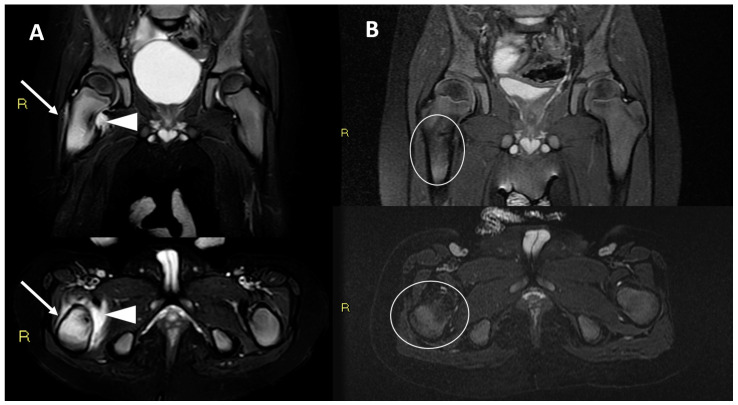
T2w fat saturated MRI images (coronal and axial) of a 27-month-old child male (at the time of the treatment), performed 2 months before (Panel (A)) and 6 months after radiofrequency ablation (Panel (B)). Before the treatment, wide bone oedema was detectable around the nidus in the right proximal femur (arrows); extra osseous soft-tissue oedema and fluid effusion were also detectable (arrowheads). A complete normalization of intraosseous and soft-tissue findings was achieved at 6-month MRI follow-up control (oval lines).
